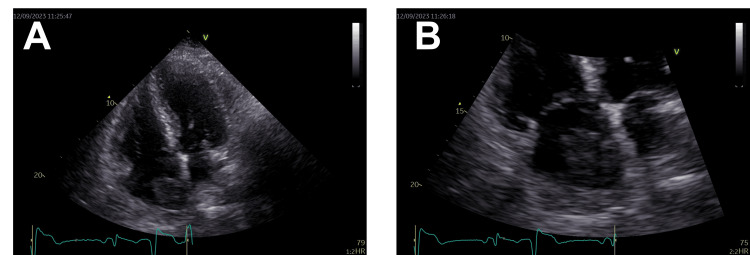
Echocardiographic imaging. A heterogeneous tissue mass (2.7 × 3.5 cm) with frond-like extensions is seen on the roof of the right atrium (RA). A larger vascular hepatic mass (5.7 × 6.2 cm) appears to be in continuity with the right atrial mass.
